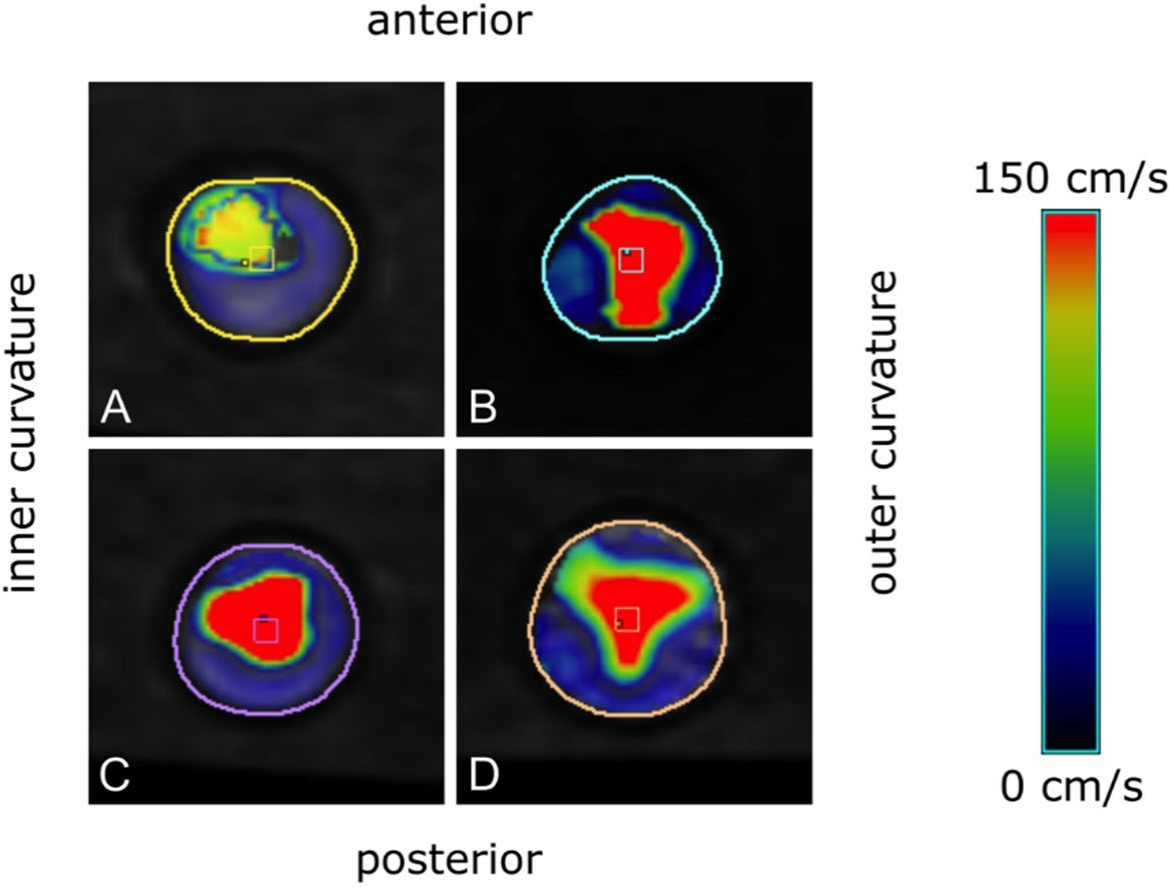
Velocity visualization within a cross-sectional plane at the *vena contracta* in 4D flow Magnetic Resonance Imaging indicating the shape of the effective orifice area: (A) INTUITY Elite 23 mm; (B) Perceval S; (C) SAPIEN 3 Ultra 23 mm; (D) Evolut PRO + 26 mm.
